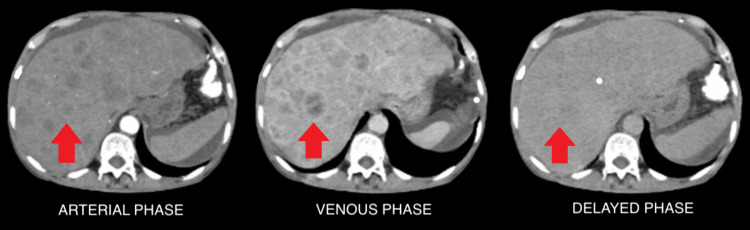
(A–C) A triple-phase CT abdomen shows multiple hypodense nodules showing peripheral enhancement on arterial and venous phases and no washout on the delayed phase in both the lobes of the liver, suggestive of dysplastic nodules.
